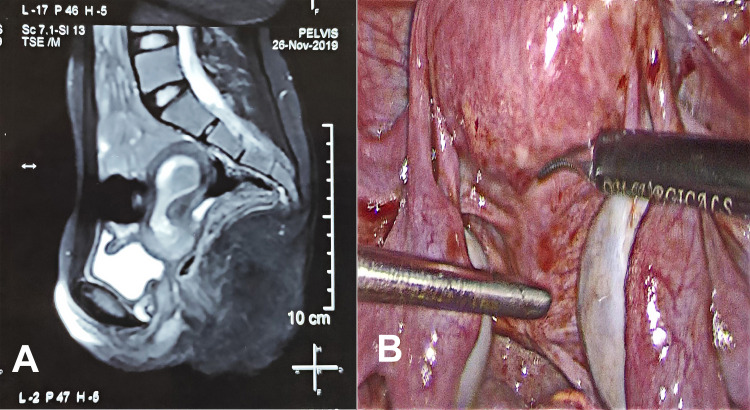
(A) MRI showing the sagittal section of the pelvis showing hematometra, cervical dysgenesis, and atresia of the proximal part of the vagina. (B) Posterior view of the cervix with focal areas of endometriosis.
